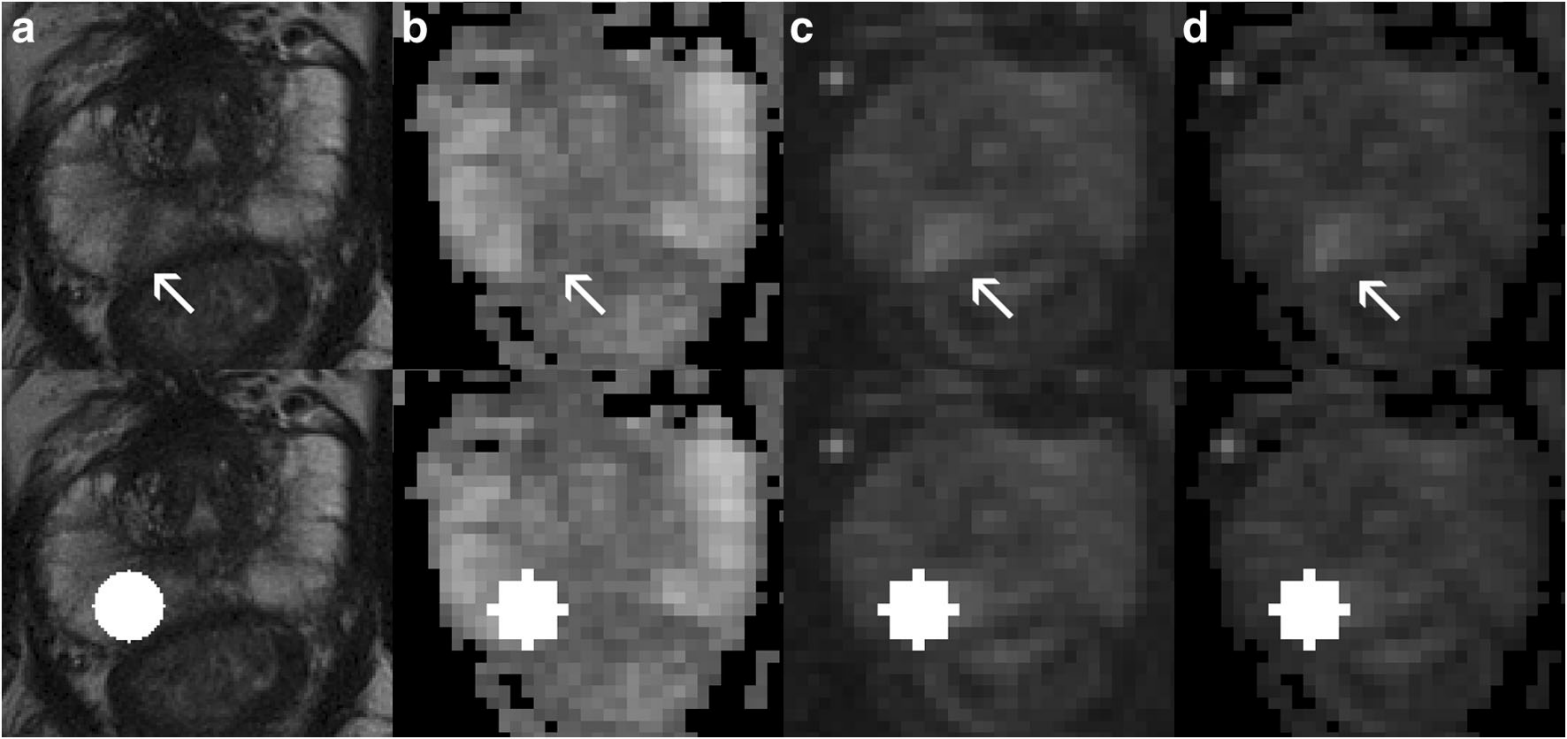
False-positive example for model 3 (T2-w + DWI) which predicted a clinically significant (CS) prostate cancer (PCa) lesion. This patient had a peripheral zone (PZ) lesion (classifiable as PI-RADS 4) which was pinpointed (the visually most aggressive part) originally by an expert (arrow, first row), which proved to be a non-CS entity (Gleason score < 6). a T2-w (axial), b ADC, c DWI *b*-value 800 s/mm^2^, d DWI calculated *b*-value 1400 s/mm^2^. Second row, segmentations using the auto-fixed volume of interest (VOI, marked in white) were placed around the visually most aggressive lesion pinpoint
